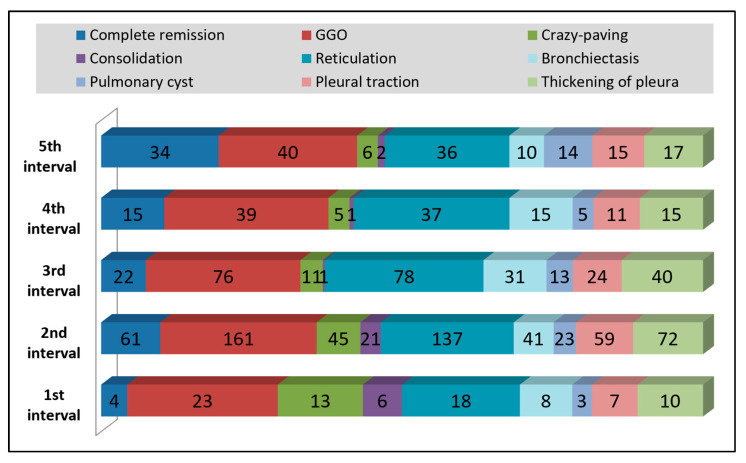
Evolution of HRCT findings by frequency in all patients included in the study.
